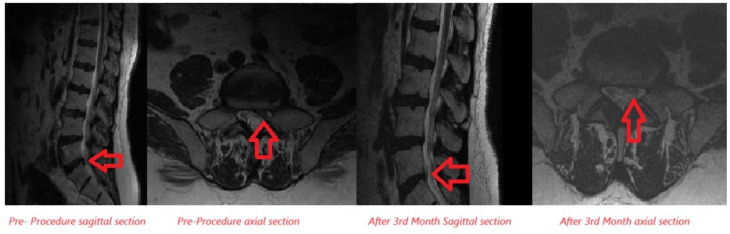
Examination of disc size reduction after ozone + PRP combine therapy in lumbar MRI sagittal and axial sections. MRI, magnetic resonance imaging; PRP, platelet-rich plasma.
